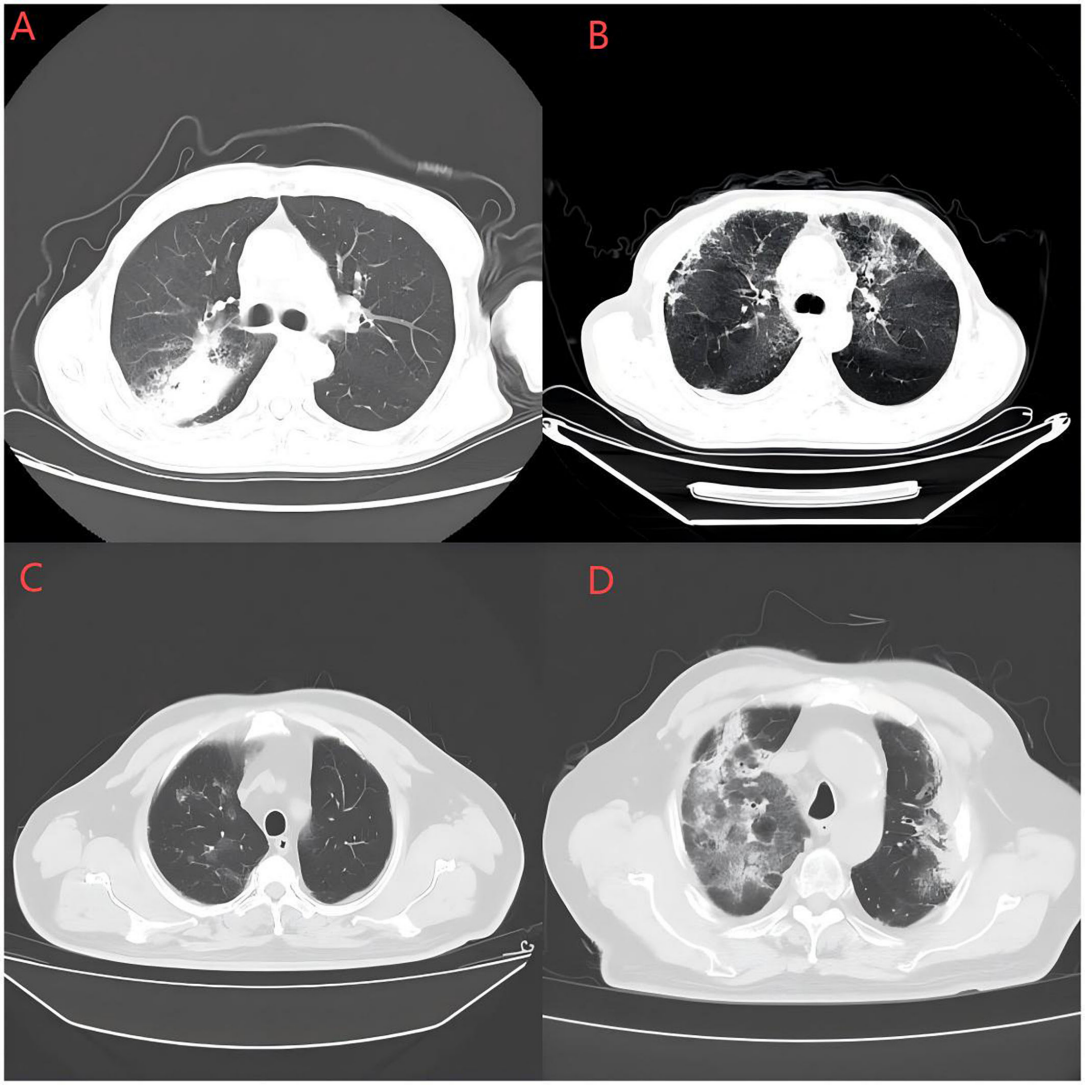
Classic CT imaging of CIP. (A) Organizing pneumonia (OP); (B) Nonspecific interstitial pneumonia (NSIP); (C) Hypersensitivity pneumonia (HP); (D) Acute interstitial pneumonia/diffuse alveolar damage (AIP/DAD).
